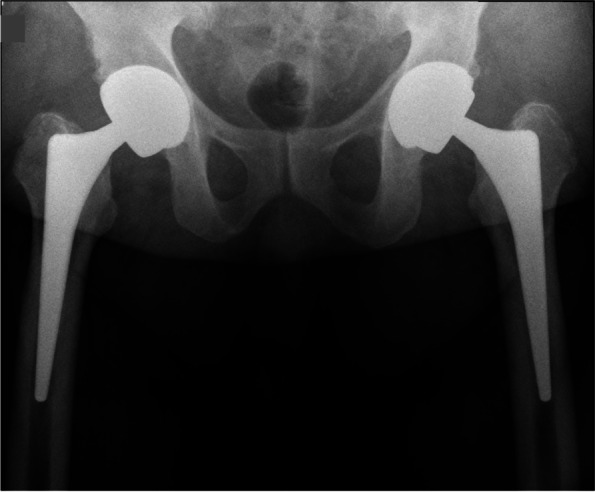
AP pelvis radiograph, 12 months after the liner dissociation on his right THA. Patient is still been pain free and prefers to pursue a conservative treatment
